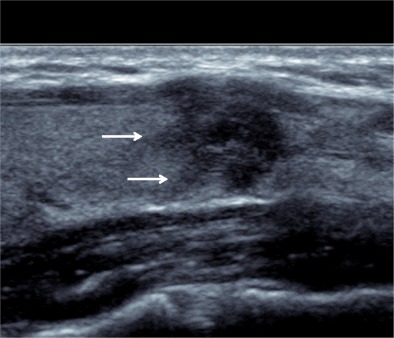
Longitudinal image of a hypoechoic nodule with a spiculated margin (*arrows*) and anterior capsular breach on high-resolution ultrasound. The lesion was confirmed as a papillary thyroid carcinoma on surgical resection
